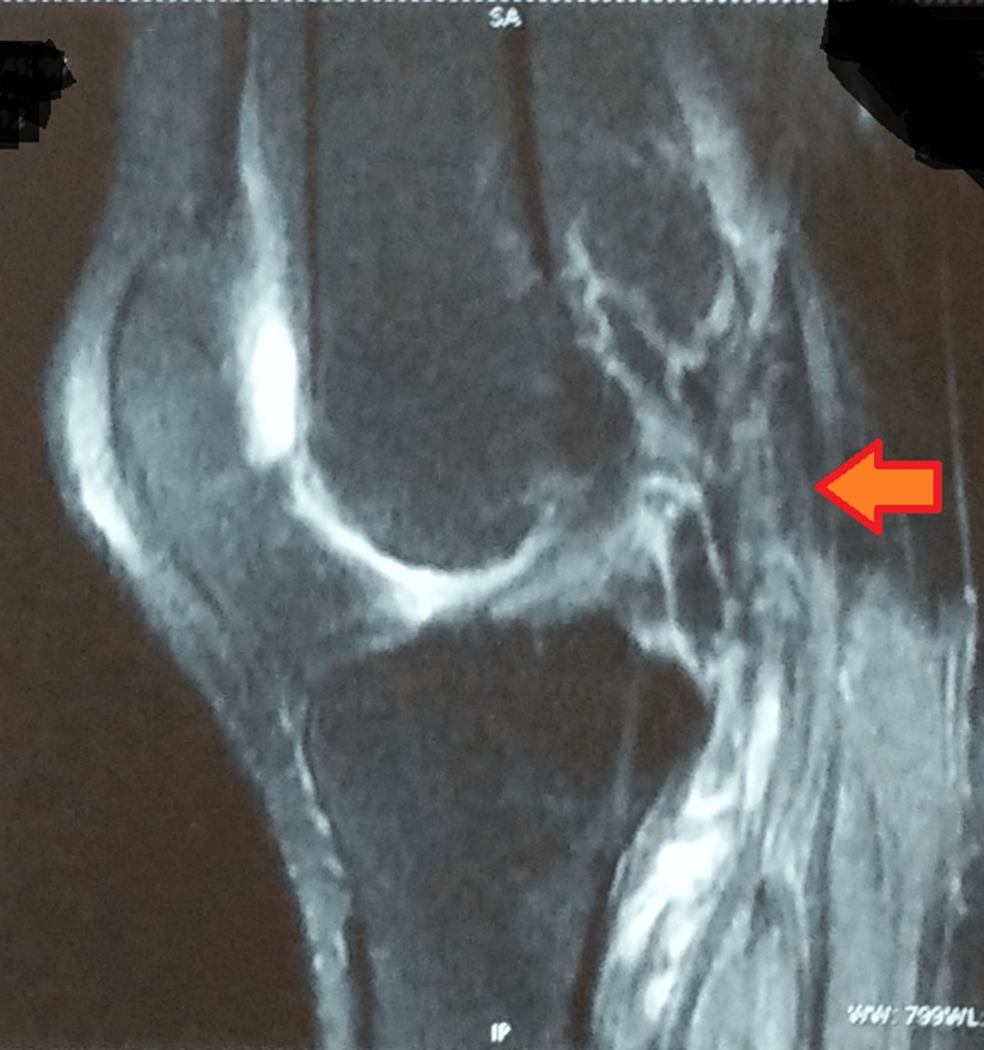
Magnetic resonance imaging without contrast-left knee joint at the six-month follow-up Arrow suggestive of joint involvement
